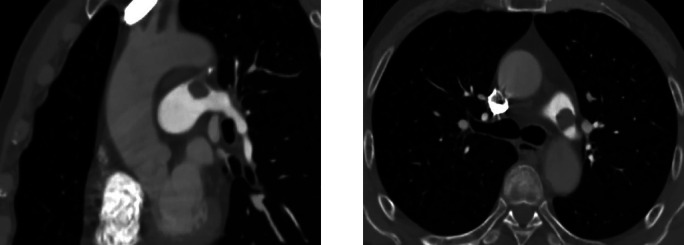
CTA of chest pain diathesis: nodular low-density filling defect in the left pulmonary artery trunk in relation to the ductus arteriosus.
